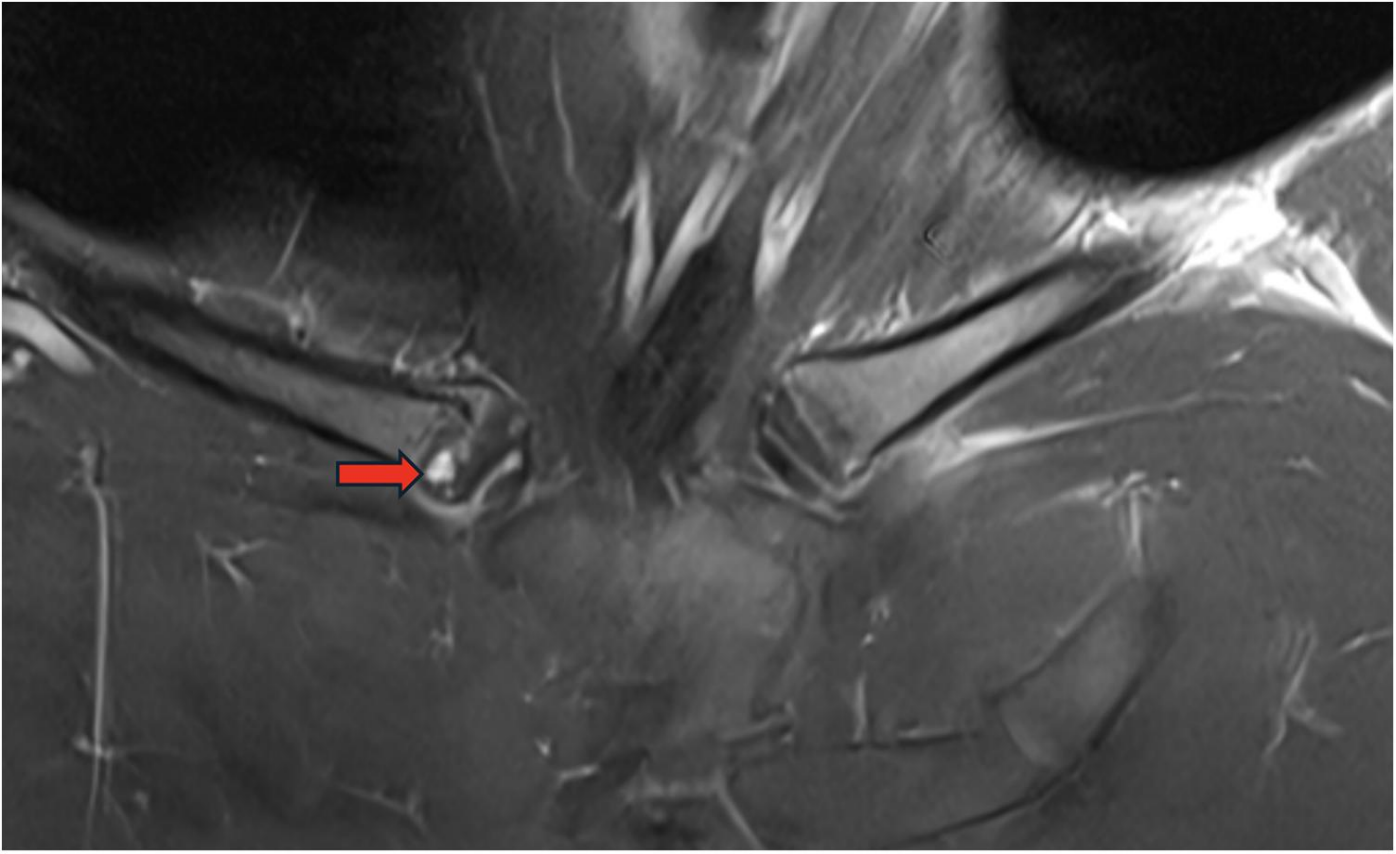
T1-weighted spin echo coronal sequence with frequency-selective fat saturation demonstrated a dysplastic appearance of the right clavicle's proximal epiphysis with a heterogeneous signal (red arrow).
